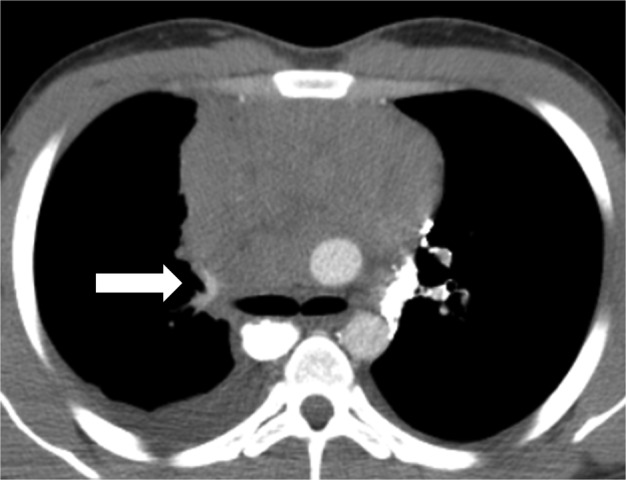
Axial view of baseline computed tomography (CT) scan that showed superior vena cava (SVC) complete collapse due to the huge seminoma mass at the height of carina (arrow).
